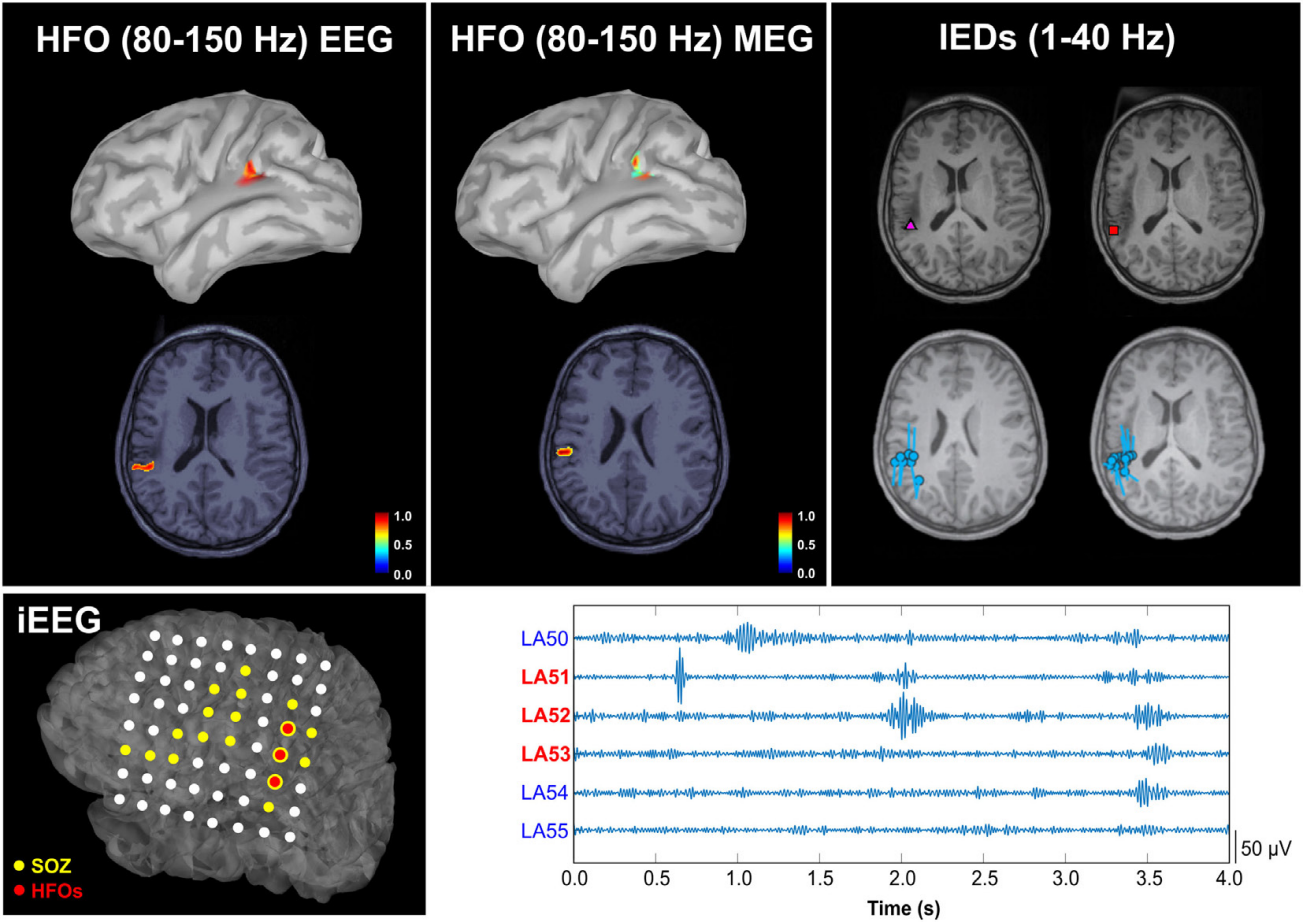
Spatial concordance between the high-frequency oscillation (HFO) zone localized non-invasively with magnetoencephalography (MEG) and high-density scalp electroencephalography (EEG), the irritative zone, and the HFO zone defined invasively with intracranial EEG (iEEG). Upper panel: localization of HFOs with scalp EEG (left) and MEG (middle), and localization of interictal epileptiform discharges (IEDs) with MEG (right) overlaid on patient’s magnetic resonance imaging (MRI). The HFO source map represents the normalized activation values thresholded at 60% of the maximum activity. Cyan circles and bars indicate the locations and orientations of the equivalent current dipoles. The purple triangle indicates the location of averaged dipoles, and the red rectangle the location of the dipoles from averaged IEDs. Lower panel: on the left, the localization of HFOs on the iEEG grid is reported (three electrodes with the highest HFO rate are highlighted in red on the map of the grid implanted on the patient’s left cortex); on the right, 2 s of data from these three channels with the highest HFO rate are shown (LA51, LA52, and LA53). The cortical location corresponding to the electrodes was determined by co-registering the post-implanted computed tomography and MRI. Source: from Papadelis et al. (51).
